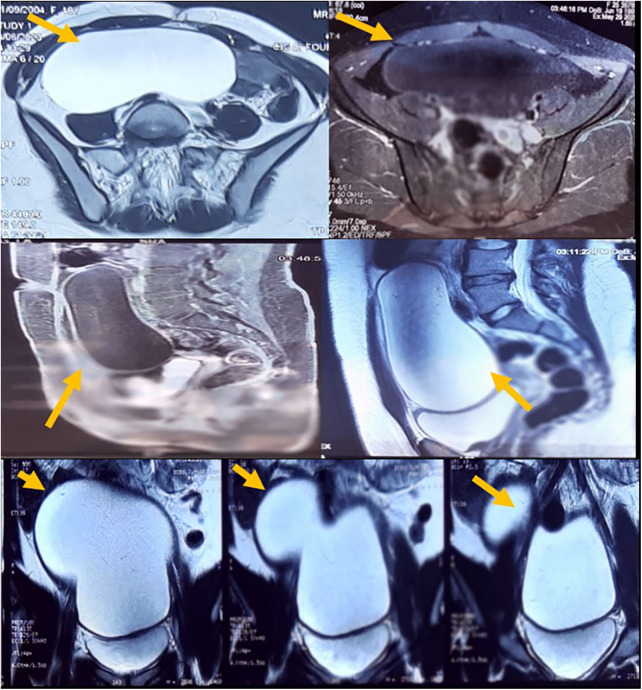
T2-weighted MRI highlights the relationship between the 280 mm cyst and the displaced uterus (yellow arrows indicate the limits of the large, unilocular cystic mass).
